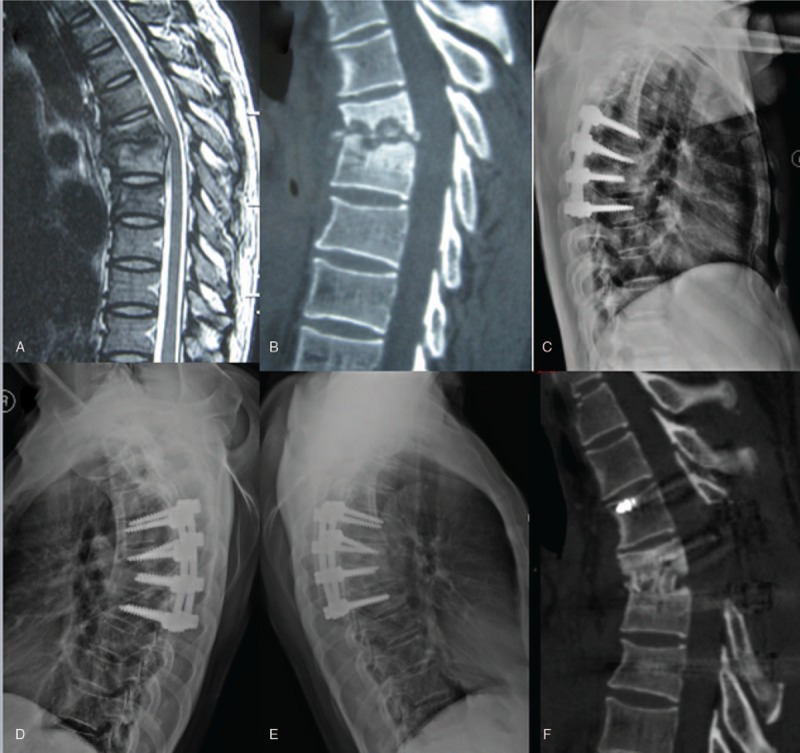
An 18-year-old man with thoracic spinal tuberculosis (T6-7) underwent posterior debridement and decompression combined with instrumentation. (A,B) Preoperative magnetic resonance imaging and CT showing bone destruction of the T6-7 vertebrae and compression of the spinal cord. (C,D) Seven-day and 6-month postoperative X-rays showing maintained correction. (EF) At 36 months’ follow-up, plain X-ray, and CT show solid bone fusion. CT = computed tomography.
